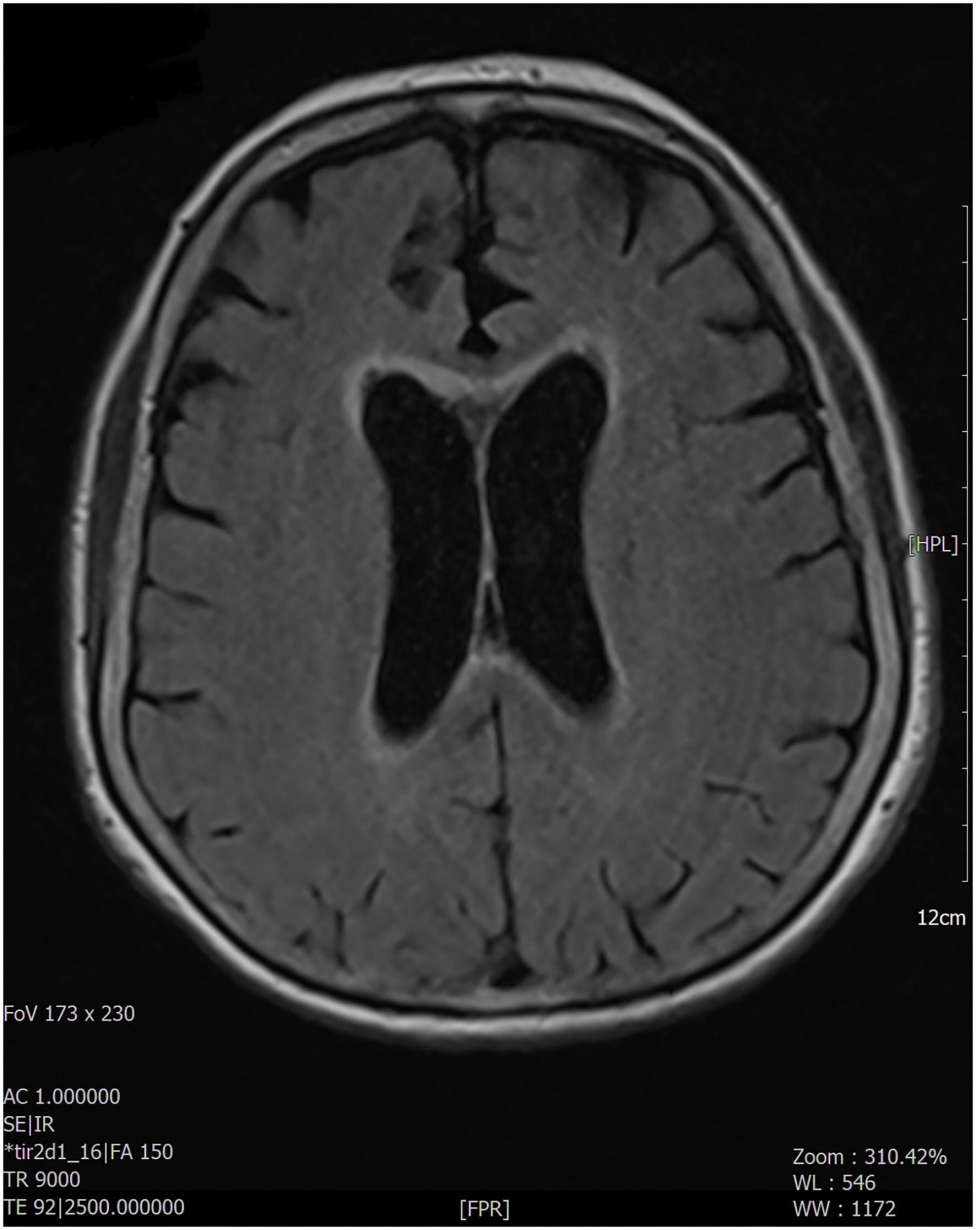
The MRI examination documented the presence, at the level of the brain, of hyperintense alteration, in FLAIR images, involving the anterior portion of the corpus callosum and the peri-ependymal white matter at the level of the lateral ventricles. The remaining brain areas of greater expression of aquaporin 4 (diencephalon, midbrain, a pons) did not appear to be affected.
